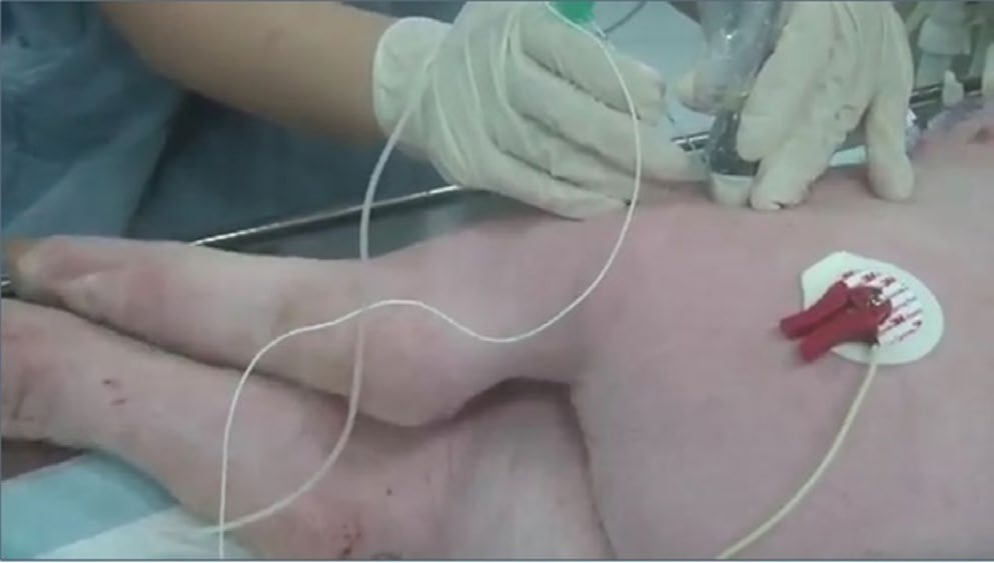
Ultrasound transducer position for midthigh sciatic nerve block, about 6 cm above the popliteal crease
